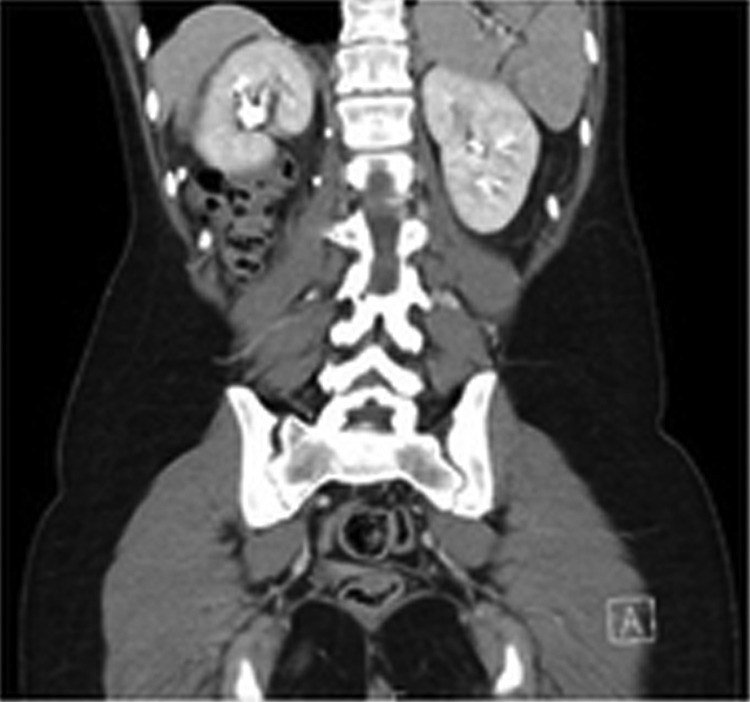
Postpartum CT demonstrating repair of the Bochdalek CDH and the appropriately positioned right kidney within the abdomen posterior to the right lobe of the liver. CDH, congenital diaphragmatic hernia.
